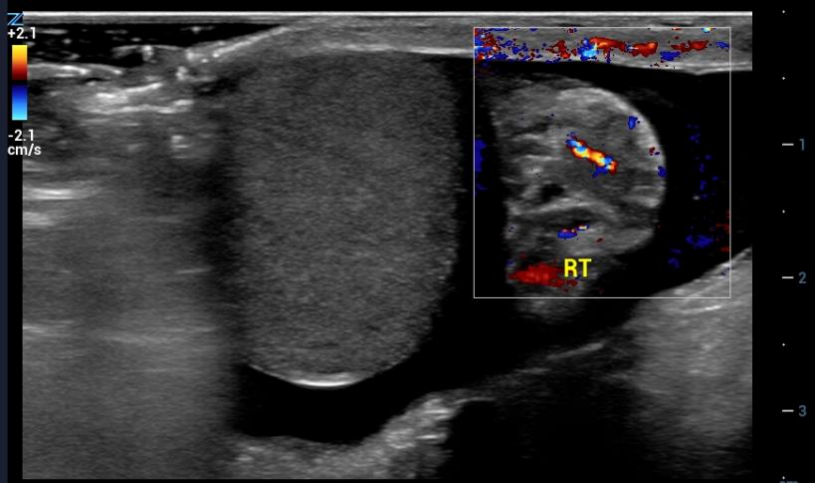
Color Doppler image with linear transducer. The testis is on the left of the color window and the circular cord complex (“whirlpool sign’) is on the right. The cord complex can be difficult to distinguish from epididymis. Here, there is very little flow in the cord complex, as can be seen in torsion. In contrast, epididymitis would present with epididymal swelling and increased blood flow.
